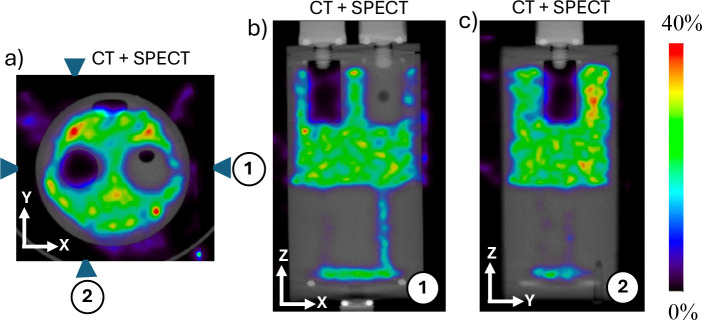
(a-c) SPECT image overlaid with CT acquired using the VETor4CT microSPECT/CT system with 79 keV and 239 keV energy windows. Two inserts, one air filled and one containing non radioactive water, are clearly distinguished from the rest volume radioactive water region.
